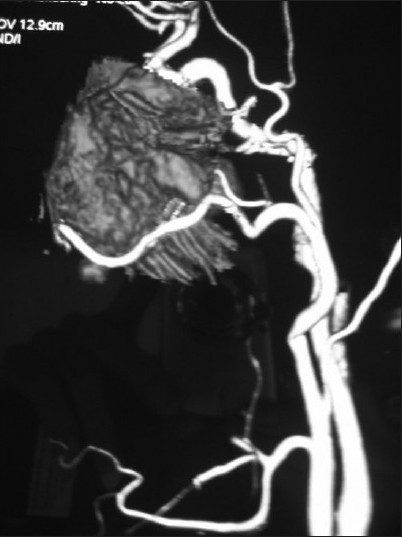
CT angiogram demonstrating normal vascular anatomy with the feeding vessels and vascularity of the tumor
